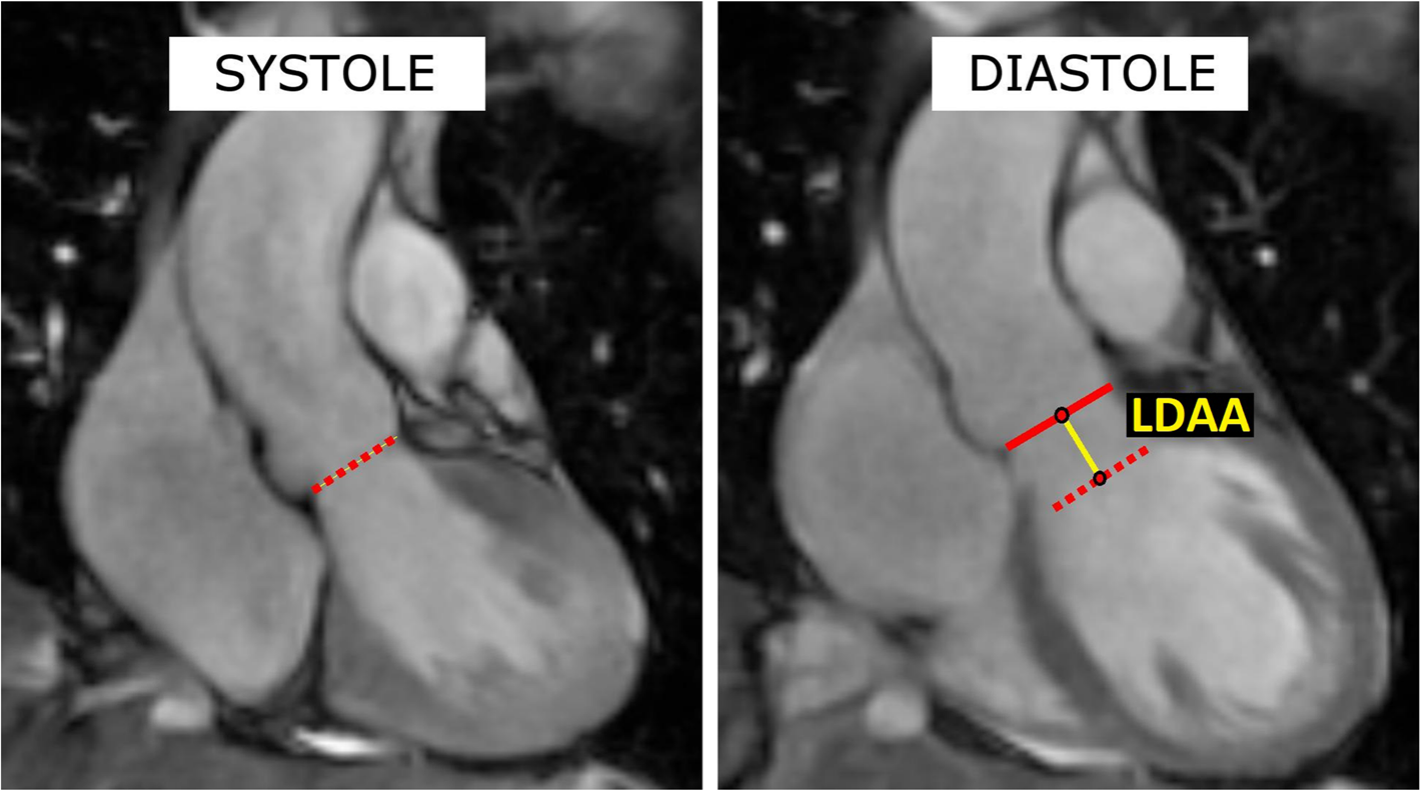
The measurements taken from the coronal cine-MRI sequences. Left (systole): red dotted line - the position of the ventriculo-aortic junction (VAJ) in systole. Right (diastole): red dotted line - the position of the ventriculo-aortic junction (VAJ) in systole, red line - position of the ventriculo-aortic junction (VAJ) in diastole; yellow line – the longitudinal displacement of the aortic annulus (LDAA)- the line links the mid-points of the VAJ in systole and diastole
